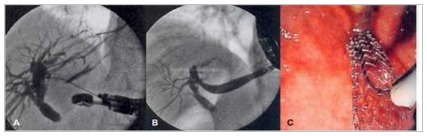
Echoguided hepatogastrostomy. A) cholangiography after ultrasound-guided
